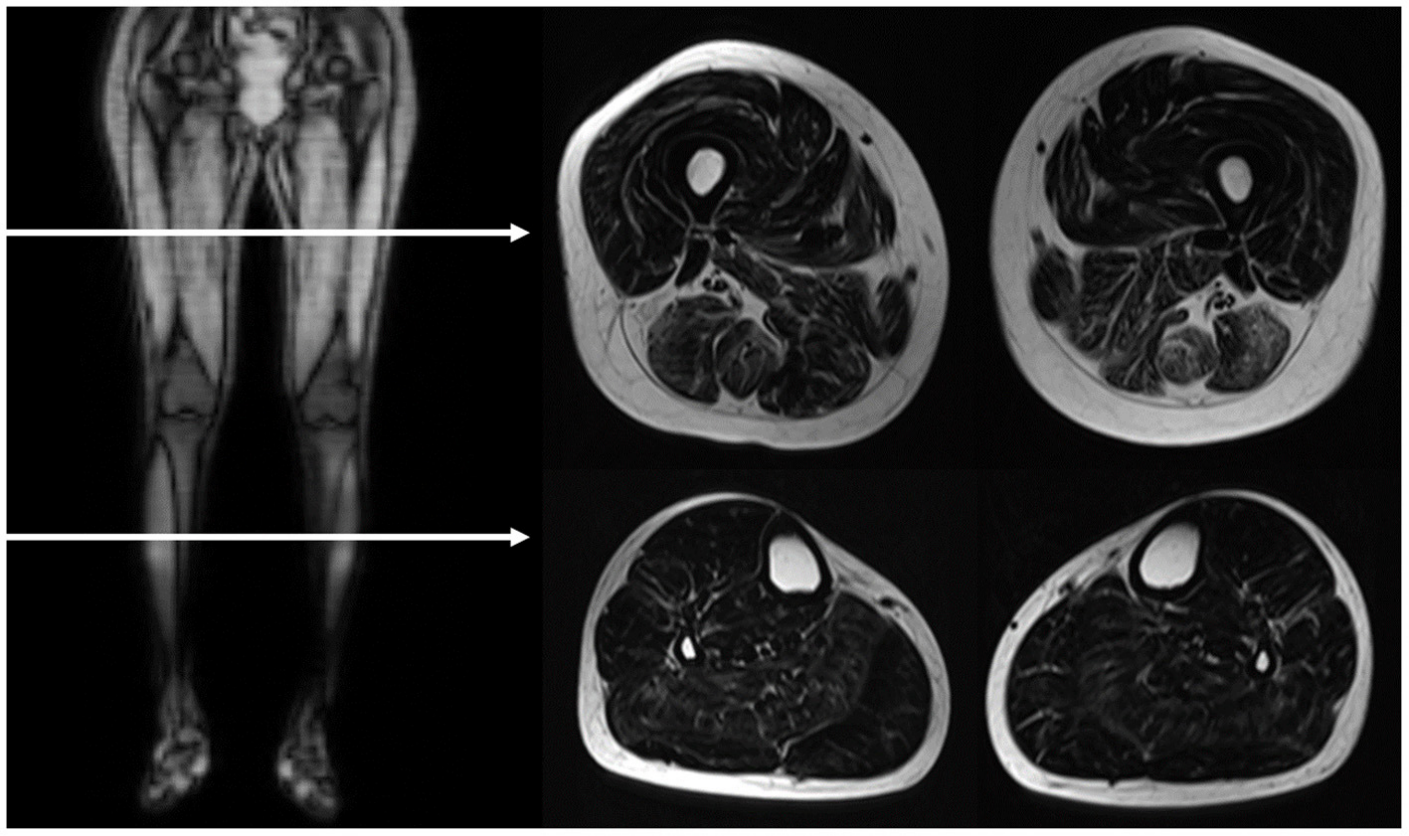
MRI image acquisition. The horizontal arrows in the right side coronal T1-weighted image demonstrated the level of assessed planes, as showed in the left T2-weighted Dixon fat-only axial images. The scanning slices are at the mid-portion of the thigh and the thickest portion of the calf.
